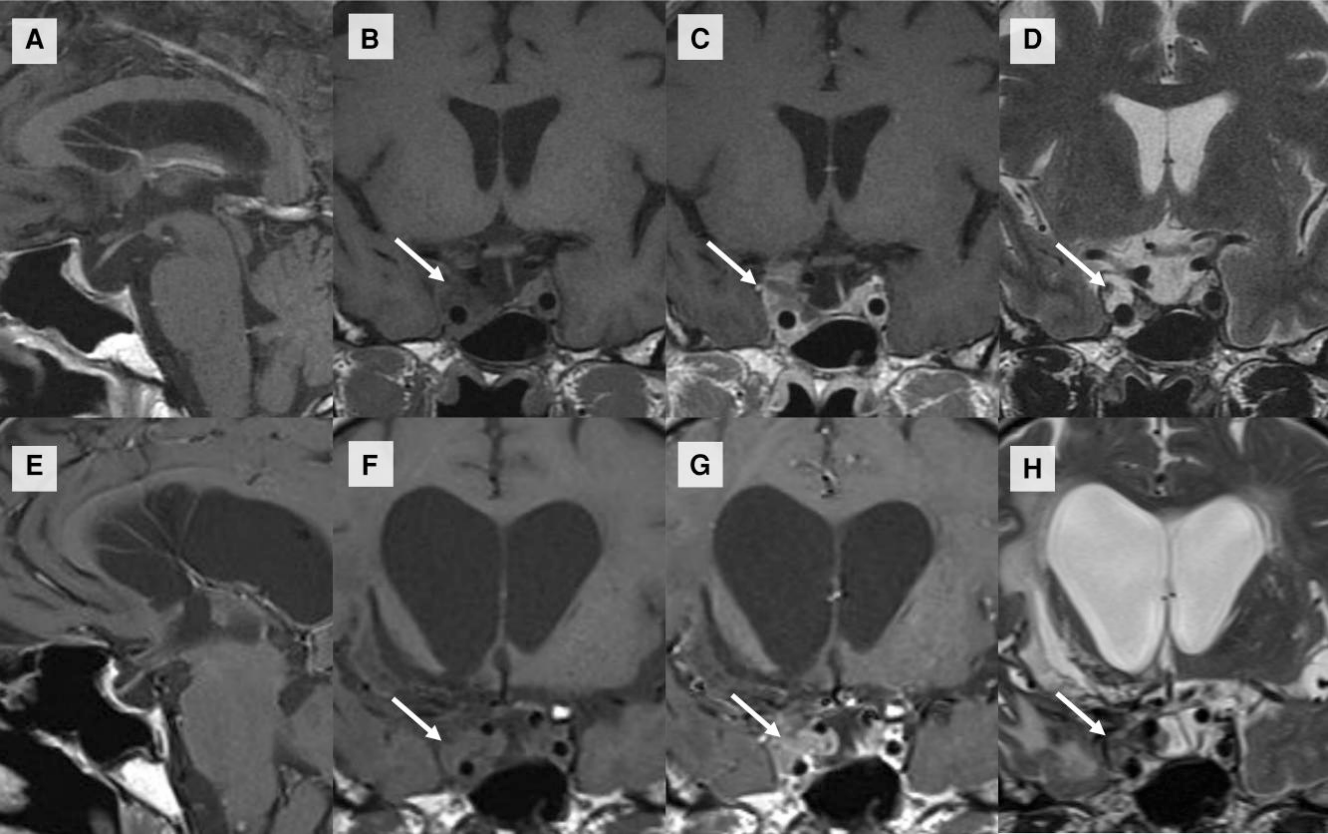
Magnetic resonance (MR) imaging of the sella turcica. Sagittal T1 (A), coronal T1 (B), coronal T1 postcontrast (C), and coronal T2 (D) images showing a residual tumor in the right cavernous sinus (arrows), predominantly isointense in T1 and hyperintense in T2, with contrast enhancement. The normal pituitary gland and pituitary stalk were displaced to the left side. Sagittal T1 (E), coronal T1 (F), coronal T1 postcontrast (G) and coronal T2 (H) images on the last MR image revealing growth of the residual tumor after radiotherapy (arrows) and a dilated third ventricle.
